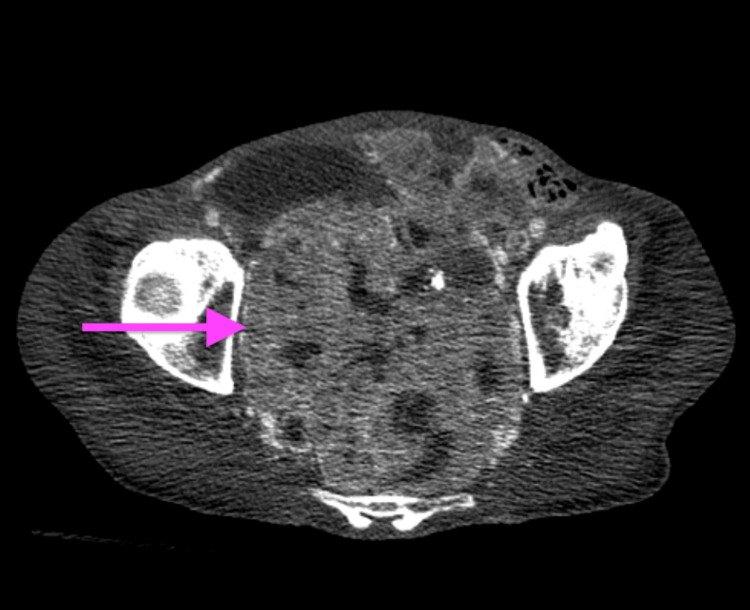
CT chest/abdomen/pelvis axial view of 15 cm x 12 cm x 15 cm heterogeneously enhancing pelvic mass
